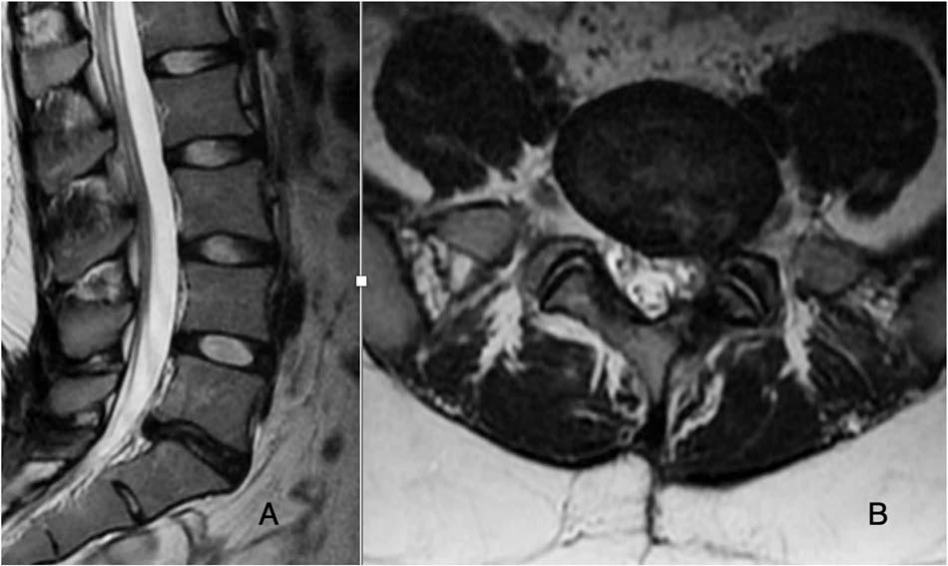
T2-weighted MRI sagittal and axial cuts demonstrate recurrent disc herniation with left-sided foraminal stenosis and degenerative changes at L5-S1.
